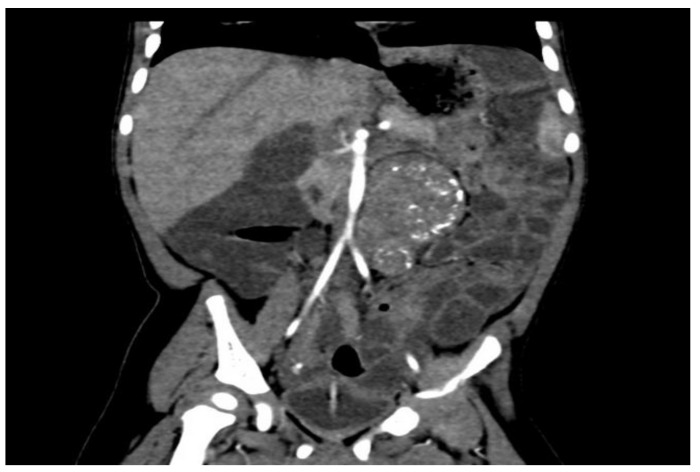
Retroperitoneal tumor with calcifications, as shown on a CT scan.
